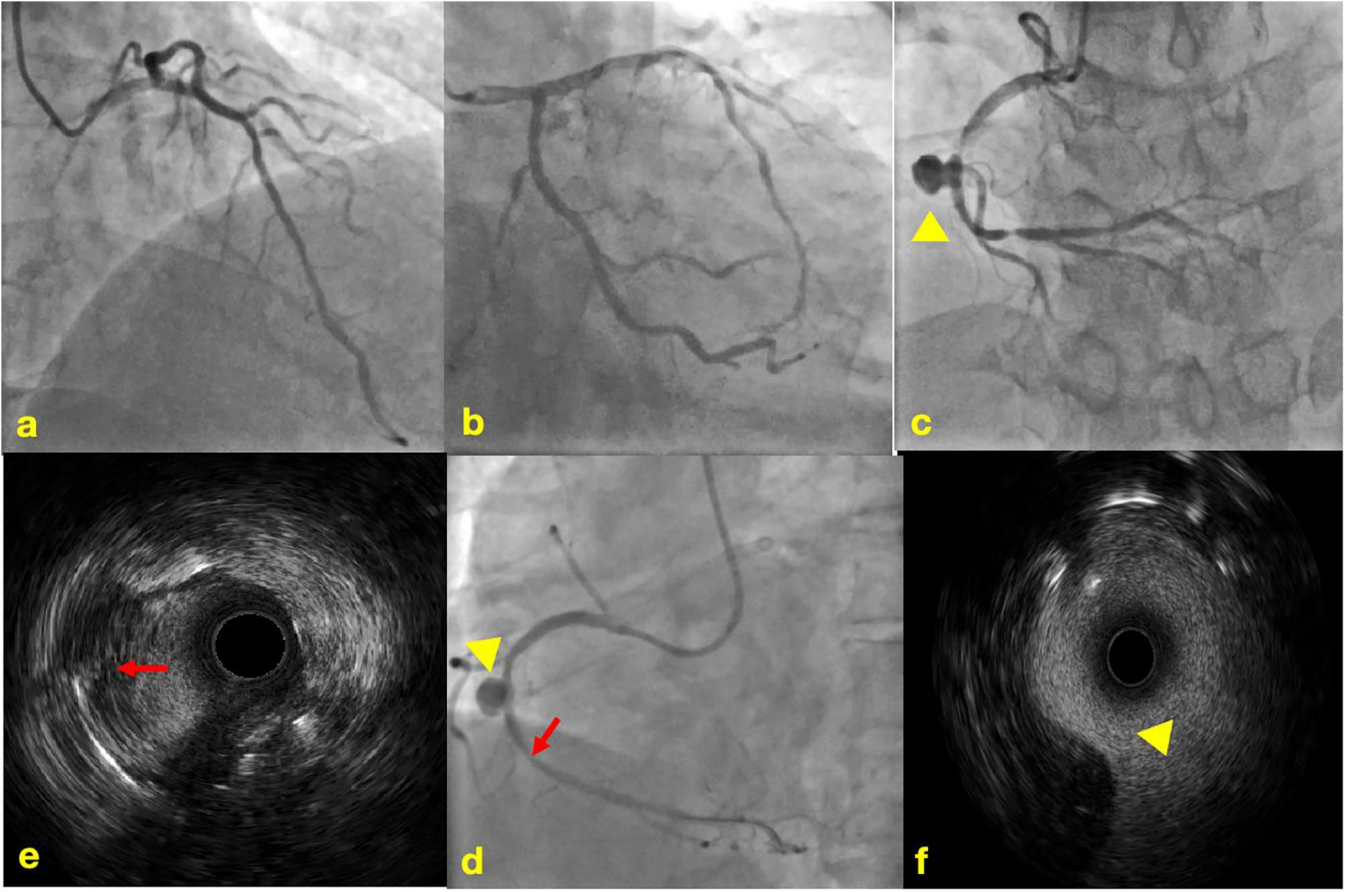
Coronary angiography and intravascular ultrasound. (a) Normal imaging of the LAD stent; (b) normal imaging of the LCX stent; (c) imaging of in-stent restenosis and coronary artery aneurysm in the RCA; (d) imaging of post-balloon dilation in the RCA; (e) IVUS image of newly formed atherosclerotic plaque; (f) IVUS image of coronary artery aneurysm, lacking the normal three-layer structure of arteries.
